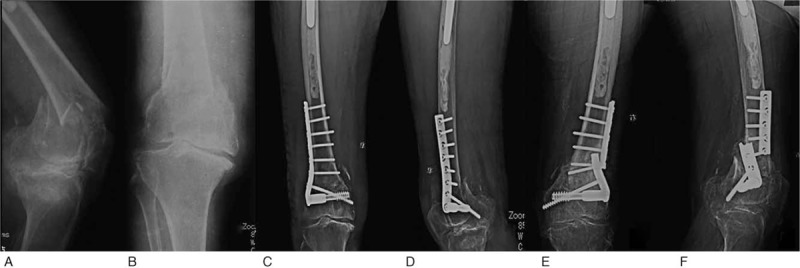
X-ray image of a female patient aged 90 years with the AO type C supracondylar femoral fracture before (A, B) and after traditional open reduction and internal-fixation (C, D). The implanted plate broke before the fracture had healed (E, F). AO = Arbeitsgemeinschaft für Osteosynthesefragen.
